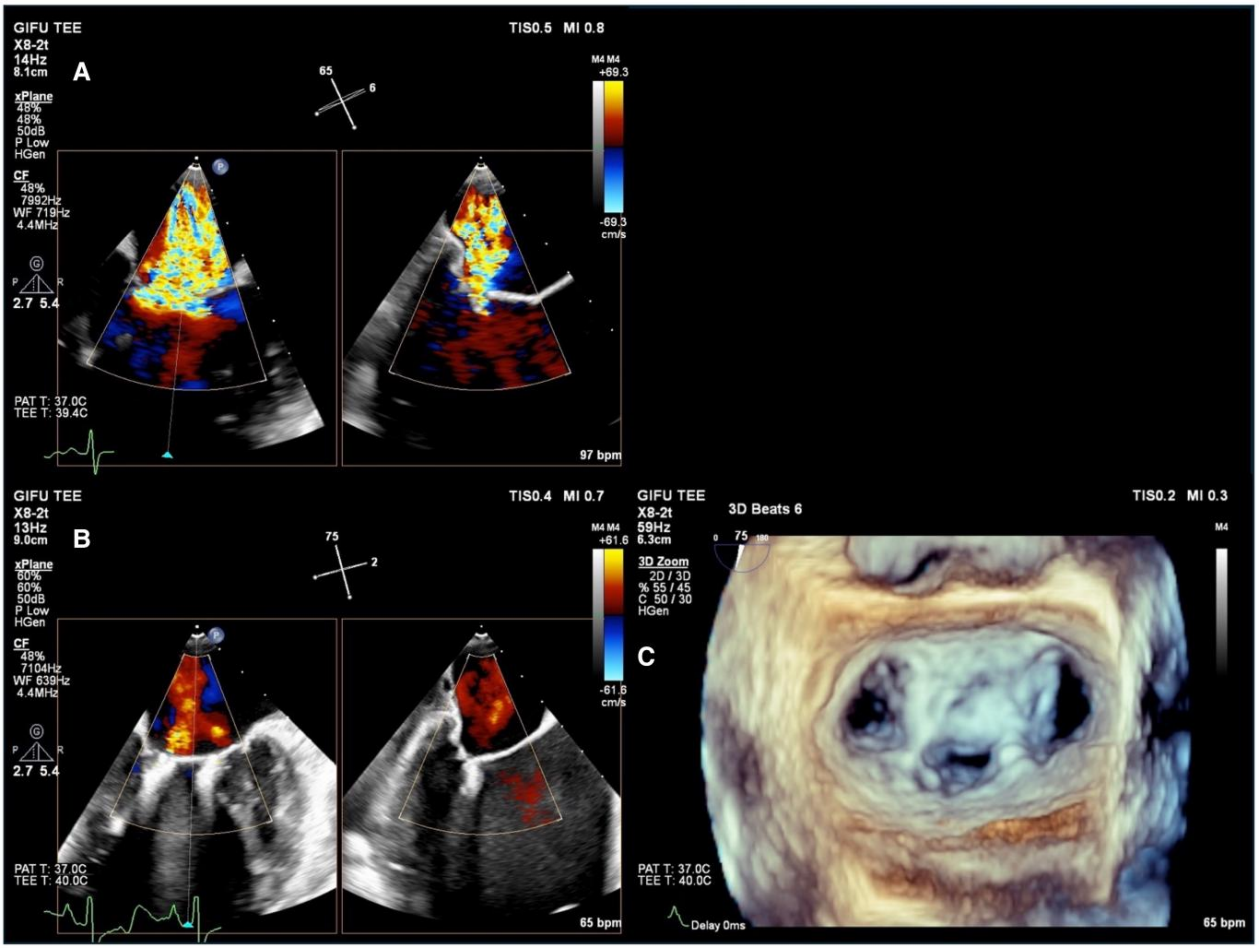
Transoesophageal echocardiography (TEE) imaging. (*A*) Pre-operative transoesophageal echocardiography imaging. (*B*) Post-procedural 2D transoesophageal echocardiography image demonstrating clip position. (*C*) Post-procedural 3D transoesophageal echocardiography image confirming final result.
